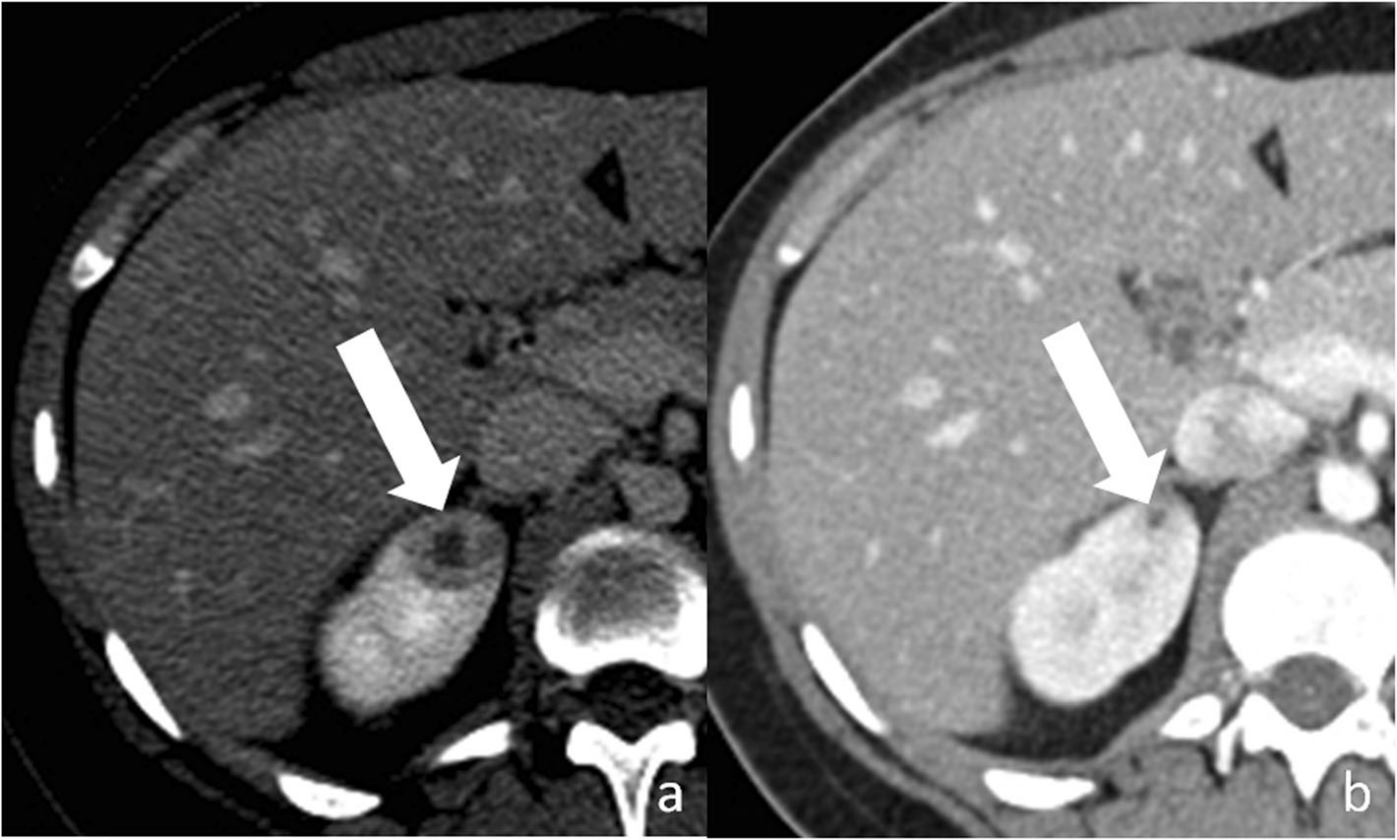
Renal abscess. a Axial contrast-enhanced CT shows a cystic lesion (arrow) with a peripheral, thick, enhancing, wall. b Axial contrast-enhanced CT obtained 3 months after antibiotic therapy shows decrease in size of the lesion
